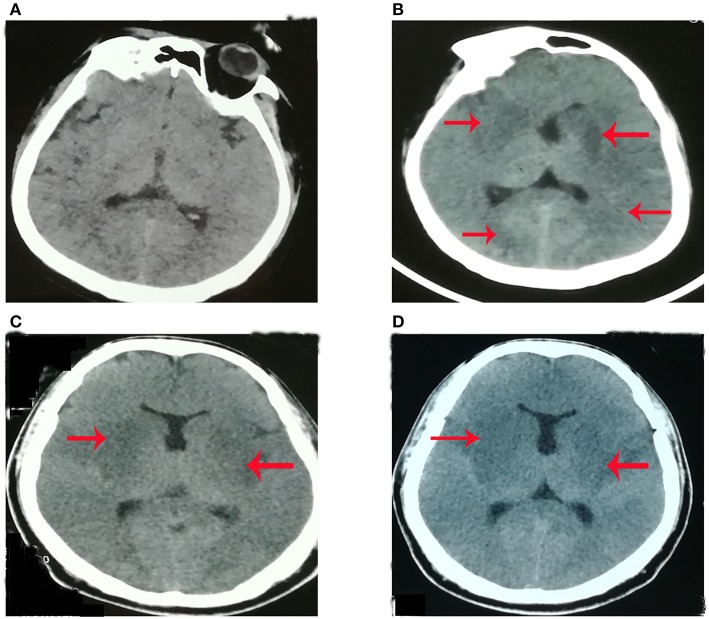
The plain brain CT imaging at different time [(A) 4th day, (B) 8th day, (C) 11th day; (D) 18th day] after initial symptom. (A) Plain CT (computer tomography) brain imaging was normal at the 4th day after initial symptoms. (B) Plain CT brain imaging showed hypo-density in the bilateral basal ganglia, bilateral occipital lobe, and left limbic lobe at the 8th day after initial symptoms. (C) Plain CT brain imaging showed hypo-density in bilateral basal ganglia, bilateral occipital lobe, bilateral limbic lobe, and left thalamic at the 11th day of initial symptoms. (D) Plain CT brain imaging showed hypo-density in bilateral basal ganglia, bilateral occipital lobe, bilateral limbic lobe, and left thalamic at 18th day of initial symptom.
